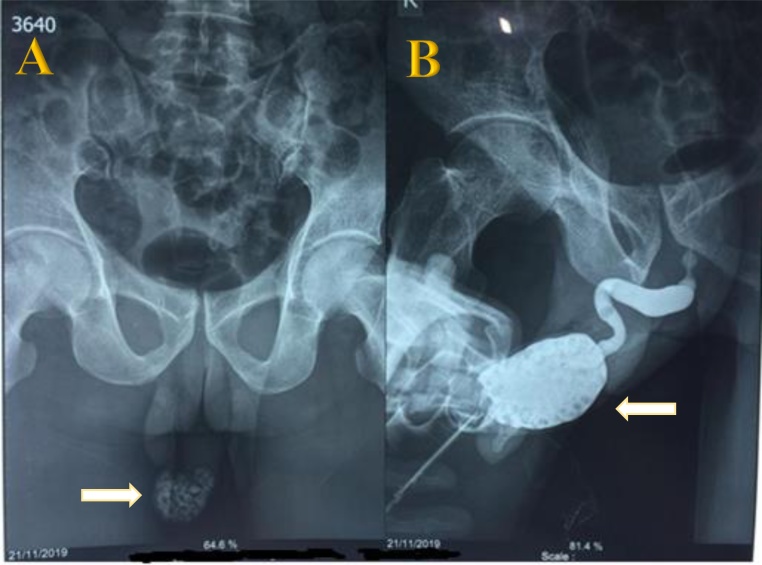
A. Plain film showing the multiple preputial stones (arrow). B. Urethrography x-ray showing multiple radio-opaque shadows in the tip of the penis (arrow), a normal caliber urethra with no evidence of stricture.
